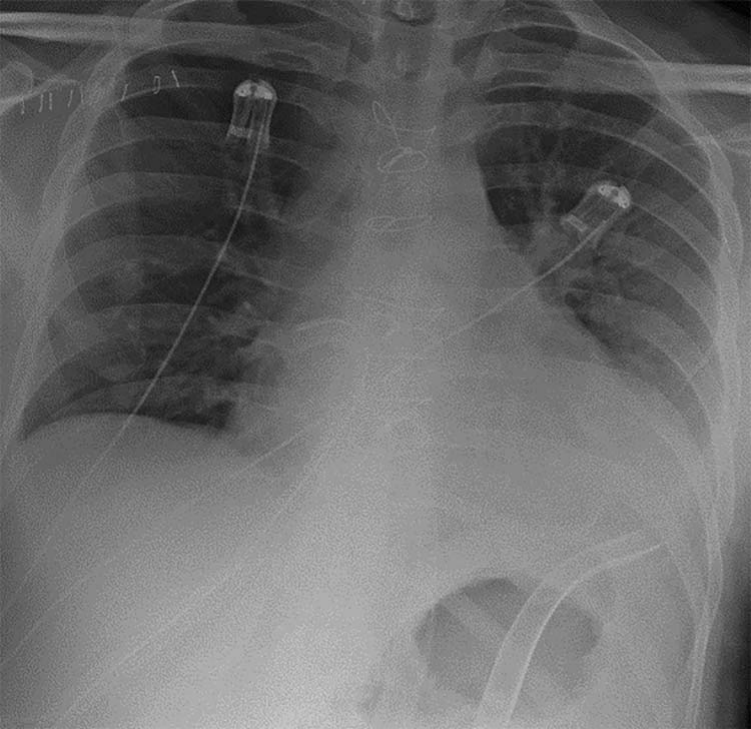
Chest radiograph demonstrating biventricular assist device pipes in situ and resolution of previous pulmonary oedema.
